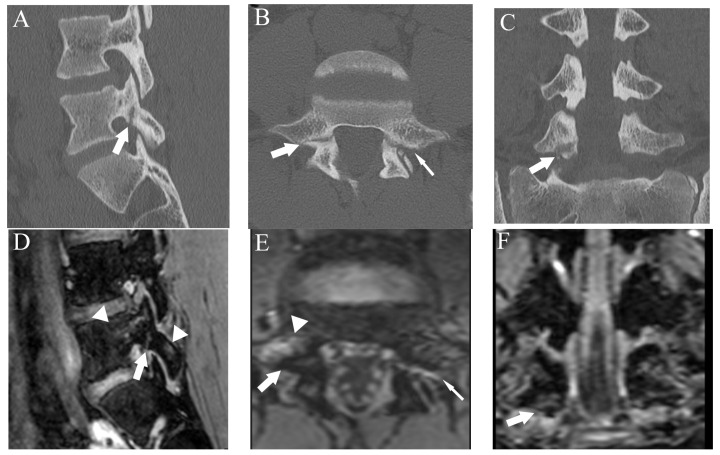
Comparison of grade 2 fracture at the right pars interarticularis at L5 from a 14-year-old male in multiple planes between CT and DESS. (A–C) CT shows grade 2 fracture on the right pars interarticularis on the right-sided sagittal, axial, and coronal plane, respectively (arrows). On the axial plane, fracture of the left side is also delineated (thin arrow). (D–F) DESS shows grade 2 fractures comparable to CT on the right-sided sagittal, axial, and coronal plane, respectively (arrows). The sagittal and axial images delineate BME around the fracture (arrowhead), and the left pars interarticularis fracture is also delineated on the axial image (thin arrow).
